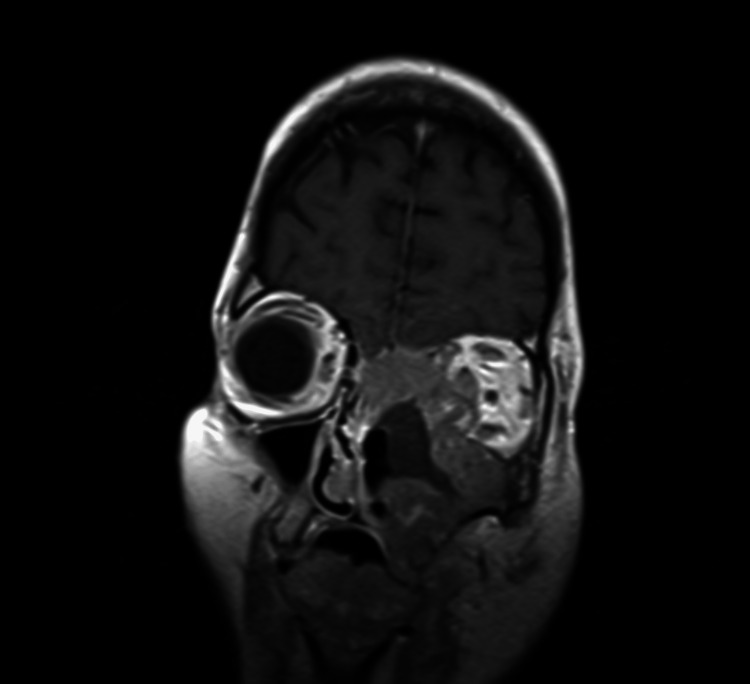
MRI brain with contrast MRI brain, coronal view of the left orbital lesion extending into the left sinonasal cavity and left preseptal tissues, nasopharynx, and left pharyngeal and masticator spaces.
